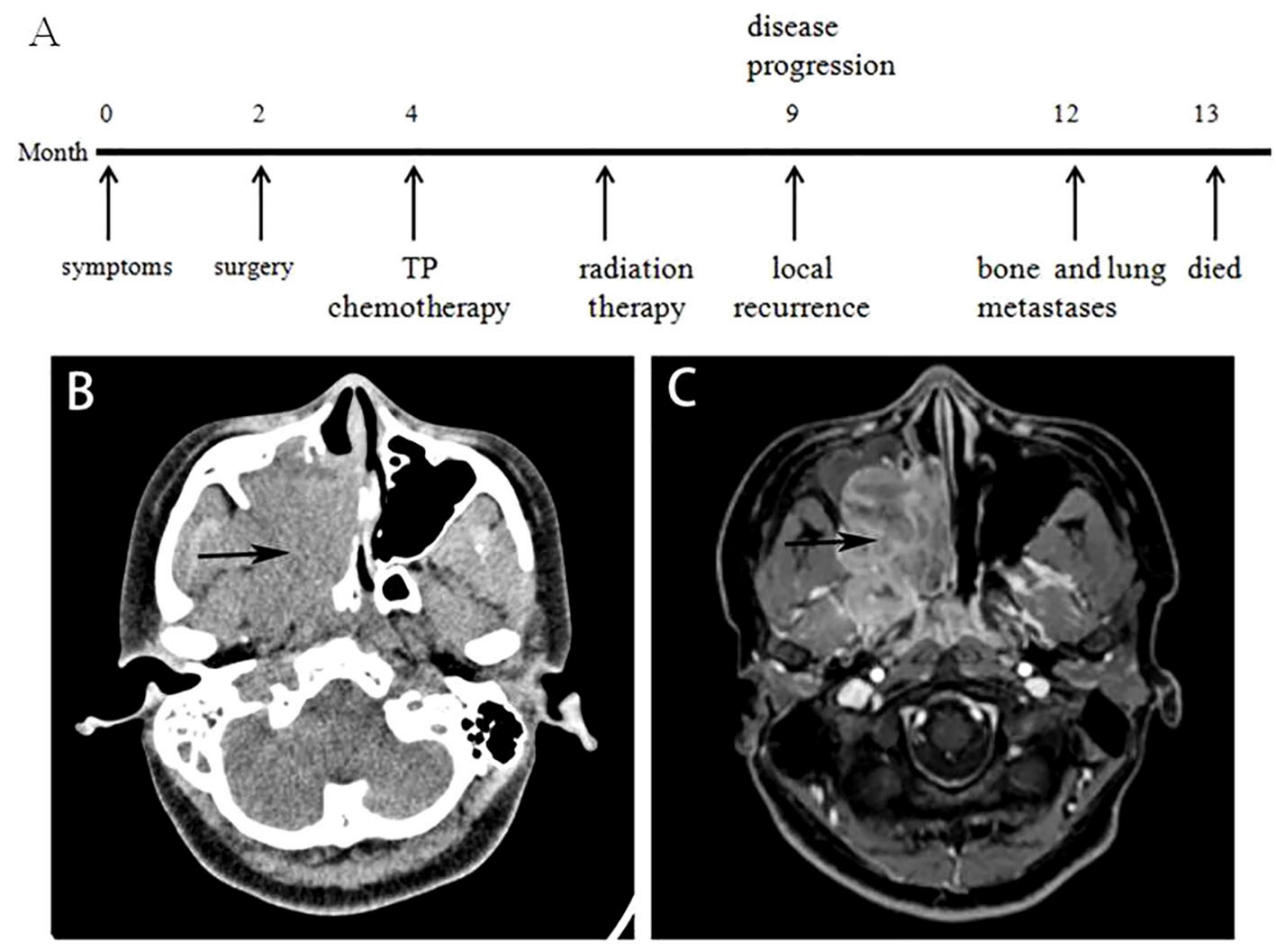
Imaging characteristics of Case 1. (A) Timeline of the diagnosis and treatment course of Case 1. (B) Contrast-enhanced CT scan of the head demonstrated a 5.1×3.1×2.3 cm sinonasal tumor filling the right maxillary sinus area and extending to the adjacent structures (black arrow). (C) Brain MRI with and without contrast demonstrating a sphenoid sinus mass.
